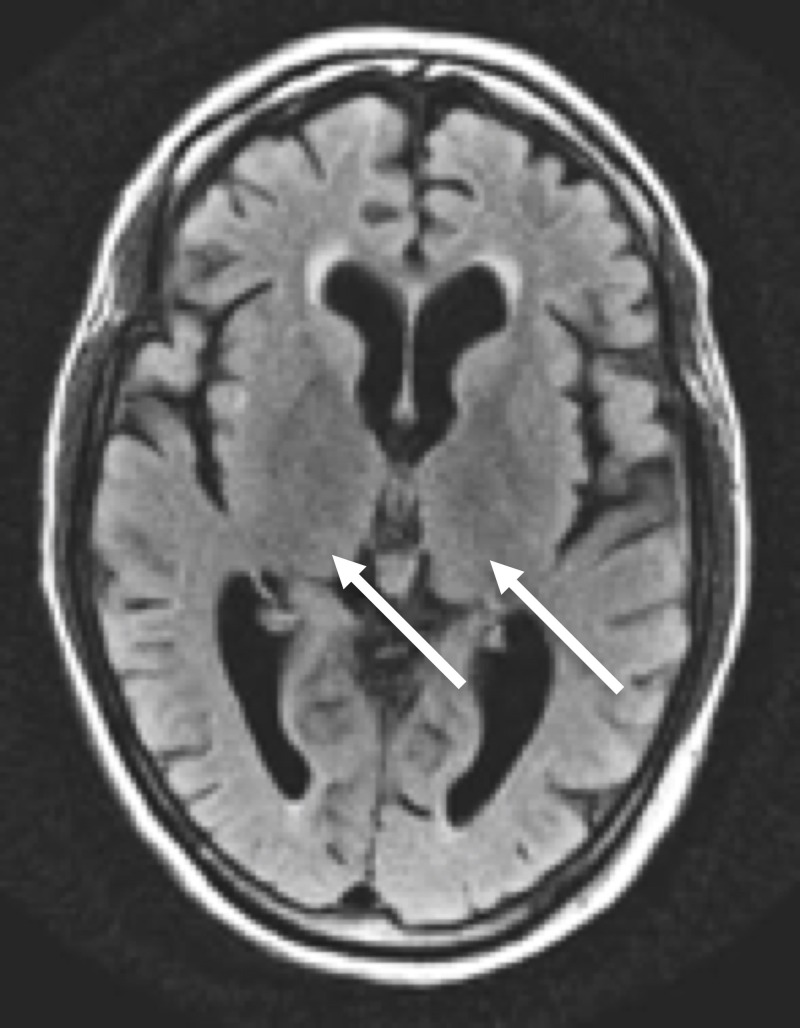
Axial FLAIR MRI of the head Axial FLAIR MRI of the head at the level of the thalami shows no significant increase in thalamic signal intensity (arrows)
